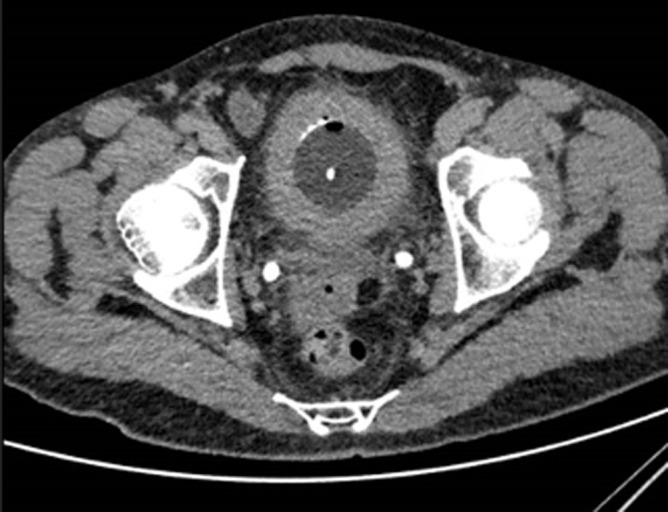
computed tomography scan showing a thick bladder wall
